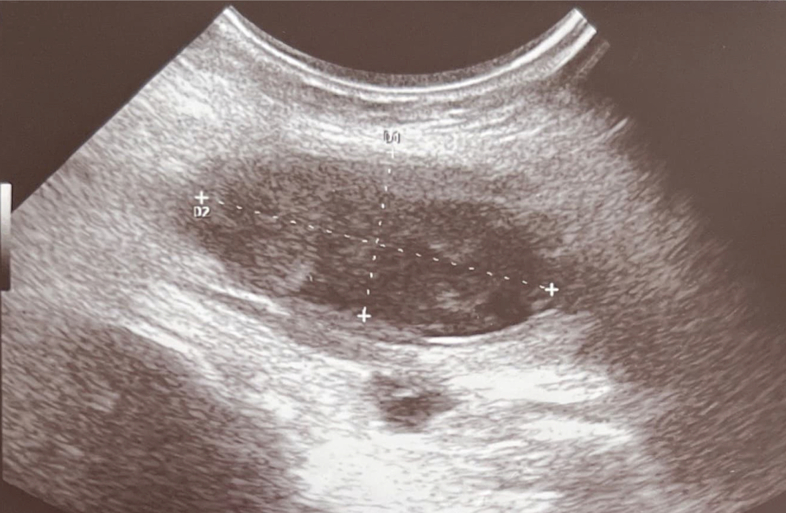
Abdominal ultrasonographic findings (longitudinal view) of a suprapubic and supravesical lesion during labor, initially considered as a colliquated myoma.
